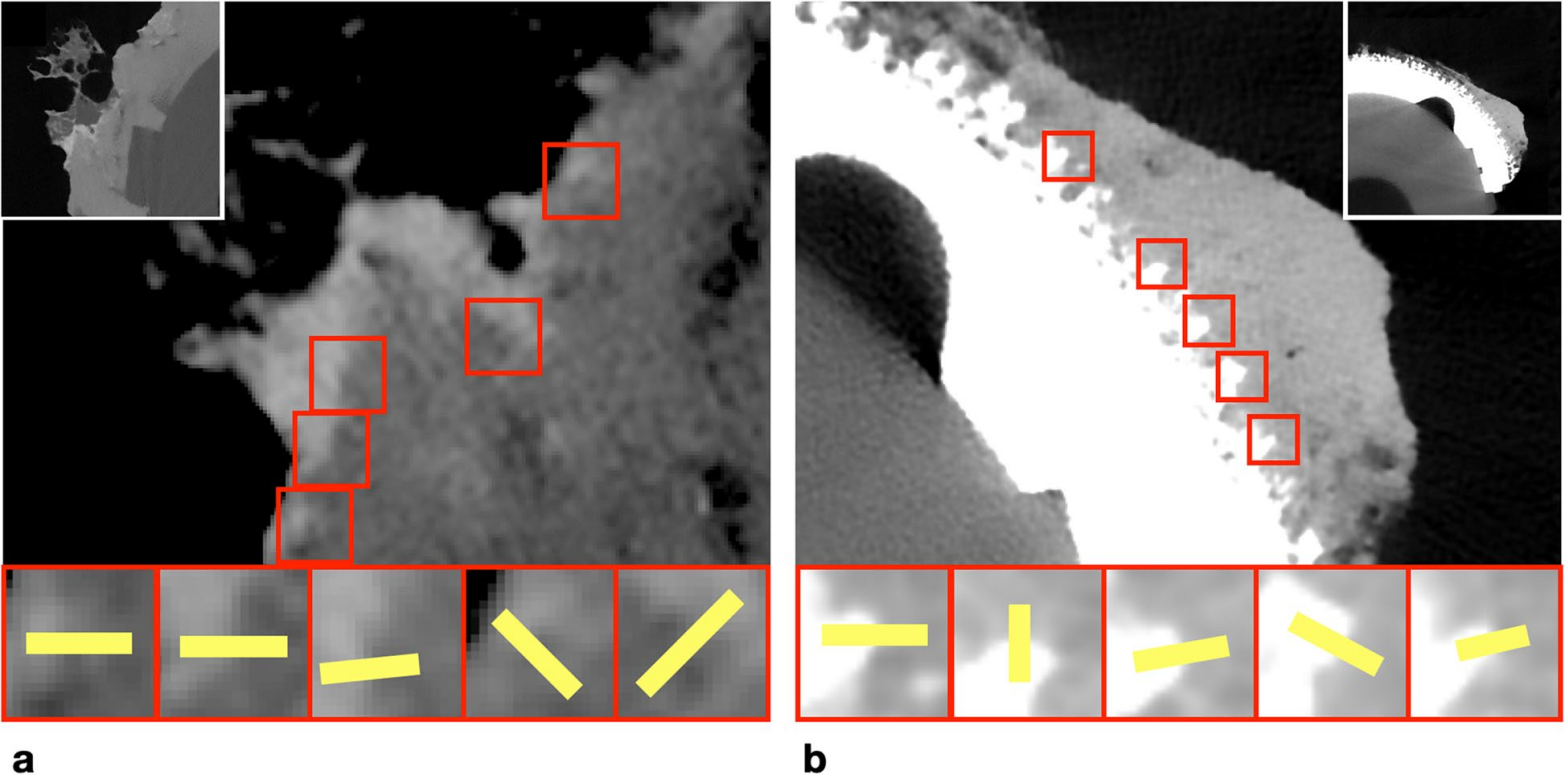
Positions of the interface sharpness measurements in the cemented cup (a) and the uncemented cup (b). Both images were obtained with photon-counting detector computed tomography, using reconstruction kernel Br98 with quantum iterative strength 4
